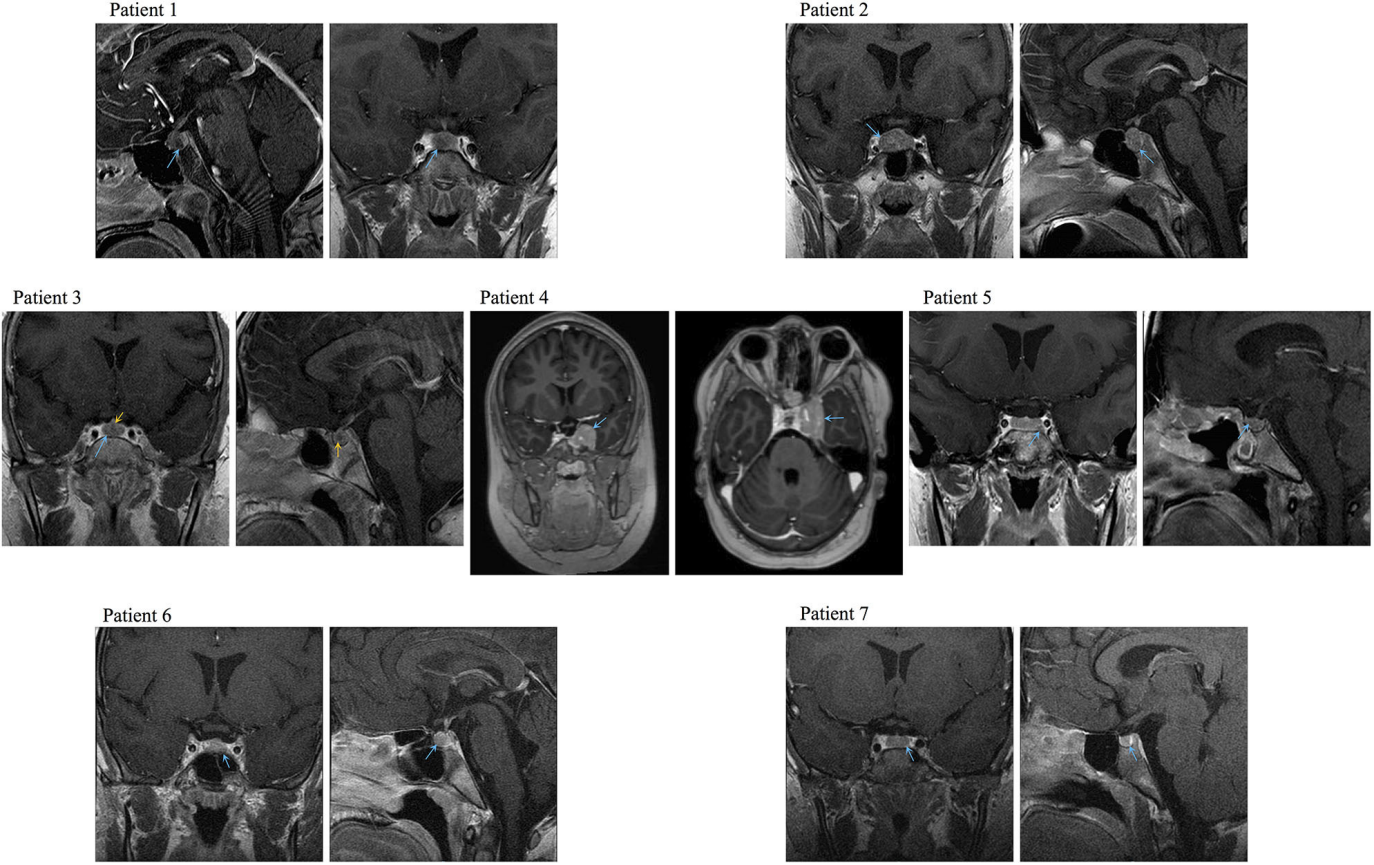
Representative preoperative MRI images of the PitNETs diagnosed in Cases 1–4, 6, and 7. Image for Case 5 was obtained after her first surgery (preoperative image not available). Blue arrows: tumors, yellow arrows: medial lobe cyst.
